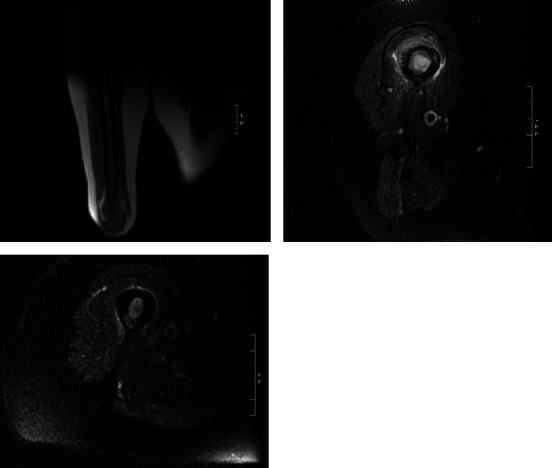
Coronal and axial magnetic resonance imaging of femur demonstrating metastatic bone disease.
